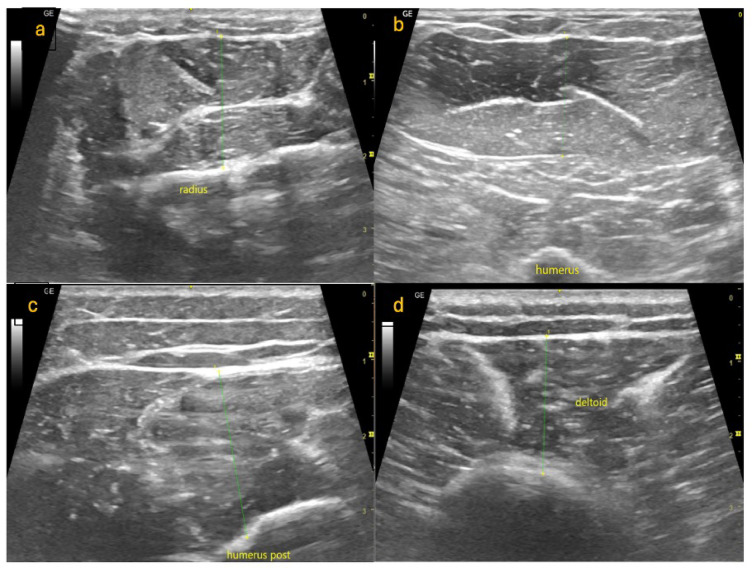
(a) Forearm, (b) Biceps Brachii, (c) Triceps Brachii, (d) Deltoid muscle thickness measurements on ultrasound image.
